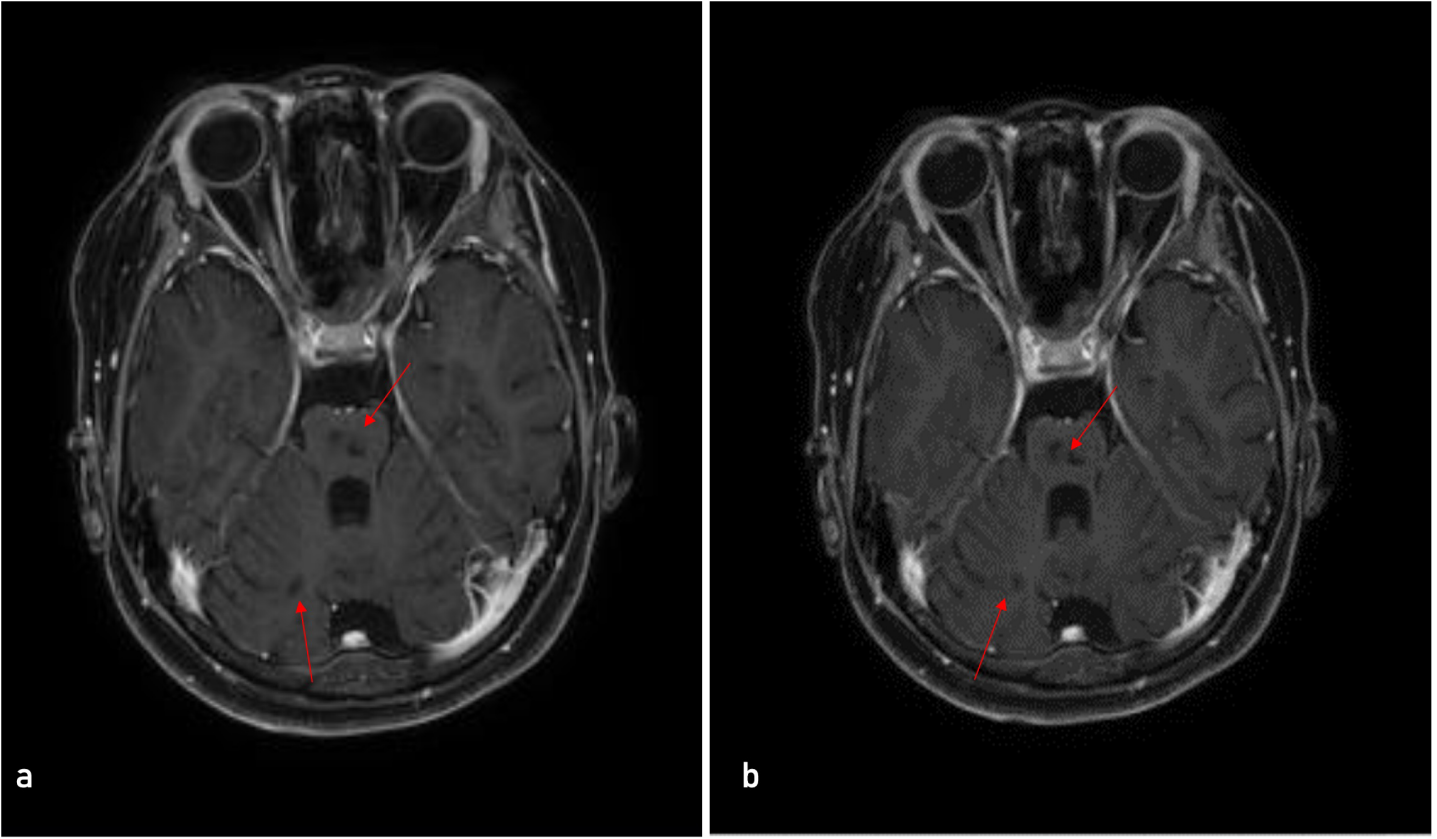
Serial axial T2-weighted images showing multiple chronic ischemic lesions with encephalomalacia. (a) Image at one level demonstrates the lesions in the bilateral cerebellar hemispheres and the pons (the area marked by the red arrow); (b) An adjacent image at another level confirms the presence and extent of the aforementioned lesions (the area marked by the red arrow).
